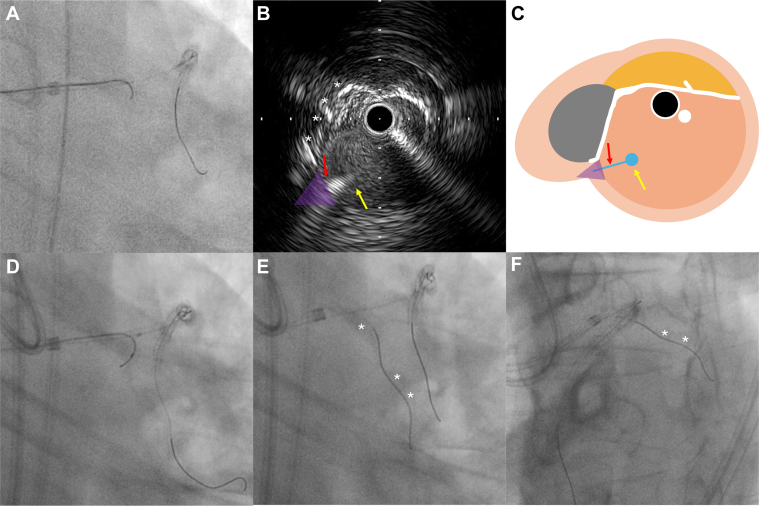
Angiographic and Intravascular Ultrasound Images When the “Move the Cap” Technique Was Performed (A) Gaia Next 4 successfully entered the extraplaque space under real-time intravascular ultrasound guidance. (B) Intravascular ultrasound image when Gaia Next 4 punctured the edge of the calcified area connecting the extraplaque space. The red arrow indicates the guidewire tip; yellow arrow, guidewire shaft; asterisk, calcification; and purple area, edge of calcification. (C) Intravascular ultrasound view. The red arrow indicates the guidewire tip; yellow arrow, guidewire shaft; white line, calcification; and purple area, edge of calcification. (D) Gladius EX traced the route that was created by Gaia Next 4. (E and F) Gladius EX following angiographic calcification. The asterisk indicates calcification.
